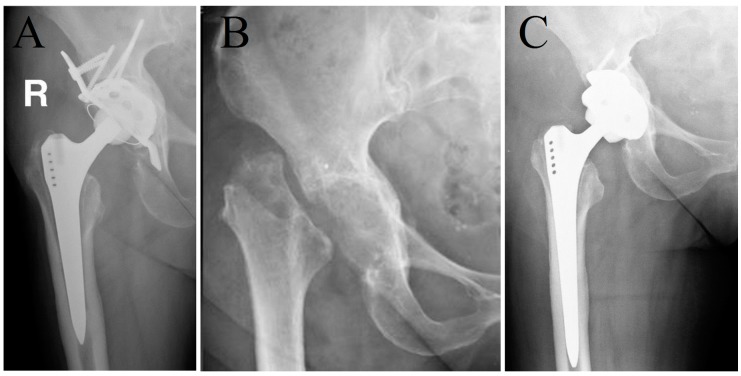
Anteroposterior radiograph of a 66-year old female patient with a periprosthetic joint infection of the right hip (A) before and (B) after the first stage (resection arthroplasty without spacer) and (C) after the second stage in a two-stage revision procedure for eradication of *Cutibacterium acnes*.
